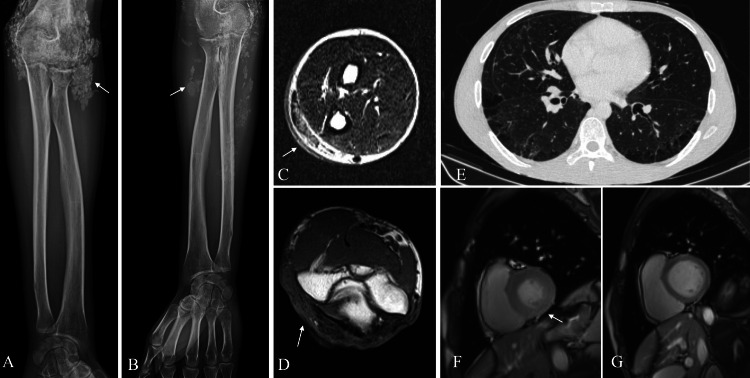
Systemic involvement of scleromyositis *A and B*: extensive calcinosis of the left and right elbow (arrows). *C*: MRI showing signs of cutaneous thickening with hypersignal of trabeculated aspect in long TR sequences (arrow). No involvement of muscle or bone planes. Small calcinosis plaques in dermis. *D:* Signal alteration of the subcutaneous cellular tissue in the form of hyposignal T1 compatible with cellulitis (arrow). Small calcinosis plaques in dermis.* E*: High-resolution CT scan showing bilateral pleuroapical thickening and paraseptal emphysema predominantly in the lower lobes and isolated areas of ground glass. *F*: Cardiac MRI 2021, diffuse myocardial inflammation with elevated T1 and T2 mapping, normal STIR with no evidence of fibrosis (arrow). *G*: Cardiac MRI 2022, no pathologic enhancements or evidence of inflammation or diffuse fibrosis.
